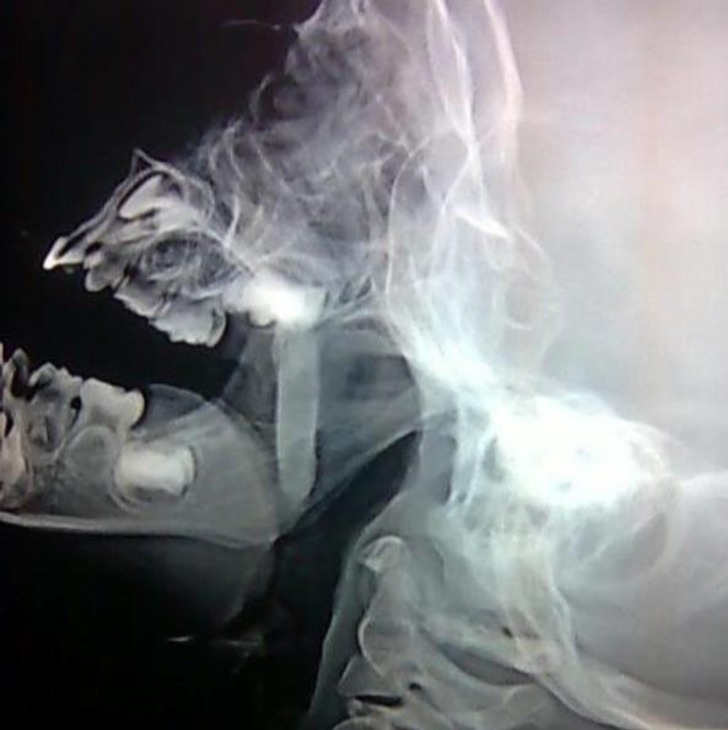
Lateral neck radiography *Nasal Endoscopy*
